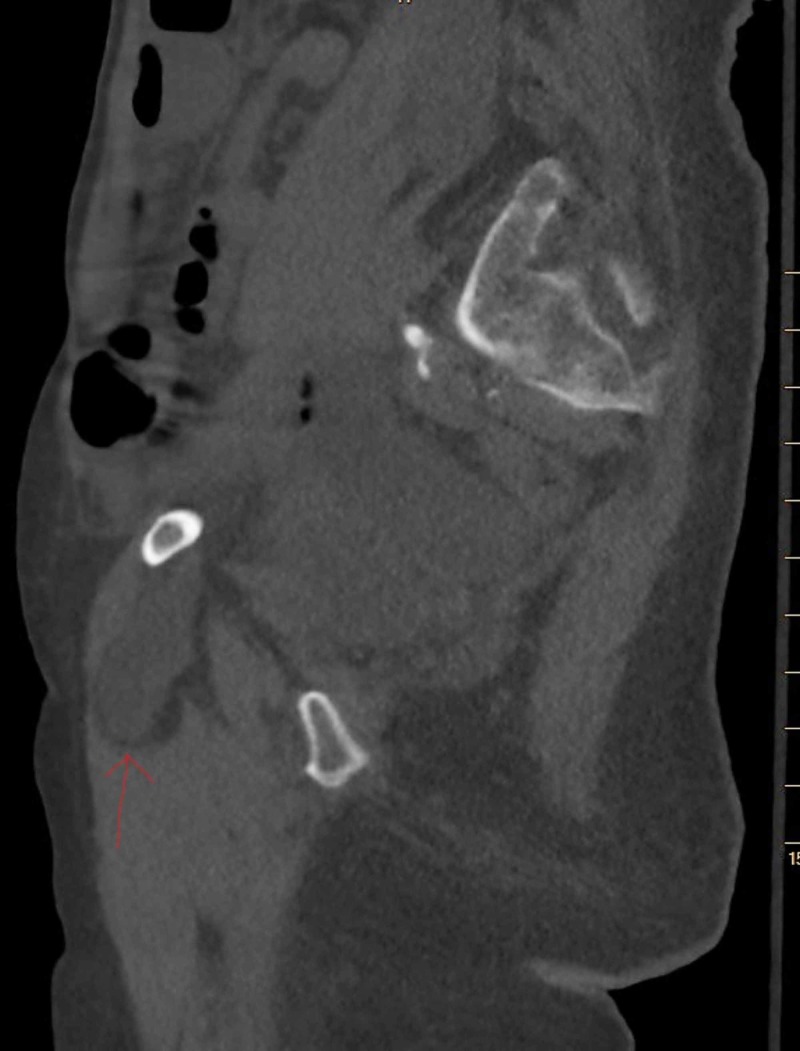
CT scan of the abdomen and pelvis showing the Right Obturator Hernia (Sagittal view).
